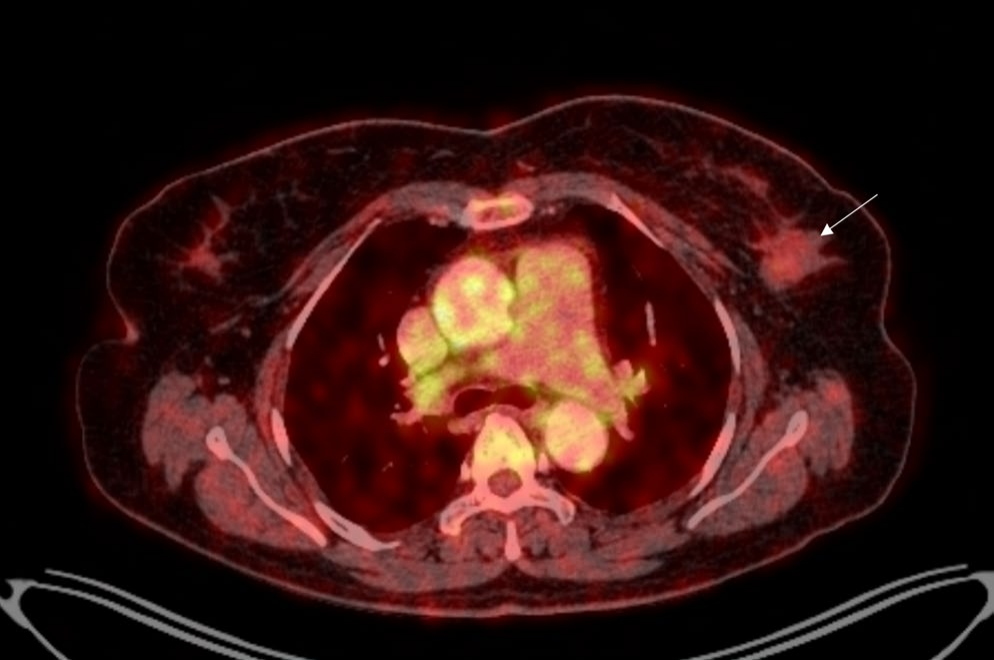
Baseline positron emission tomography/computed tomography (PET/CT) scan showing faintly FDG avid heterogenously enhancing soft tissue density lesion in the left breast (white arrow).
